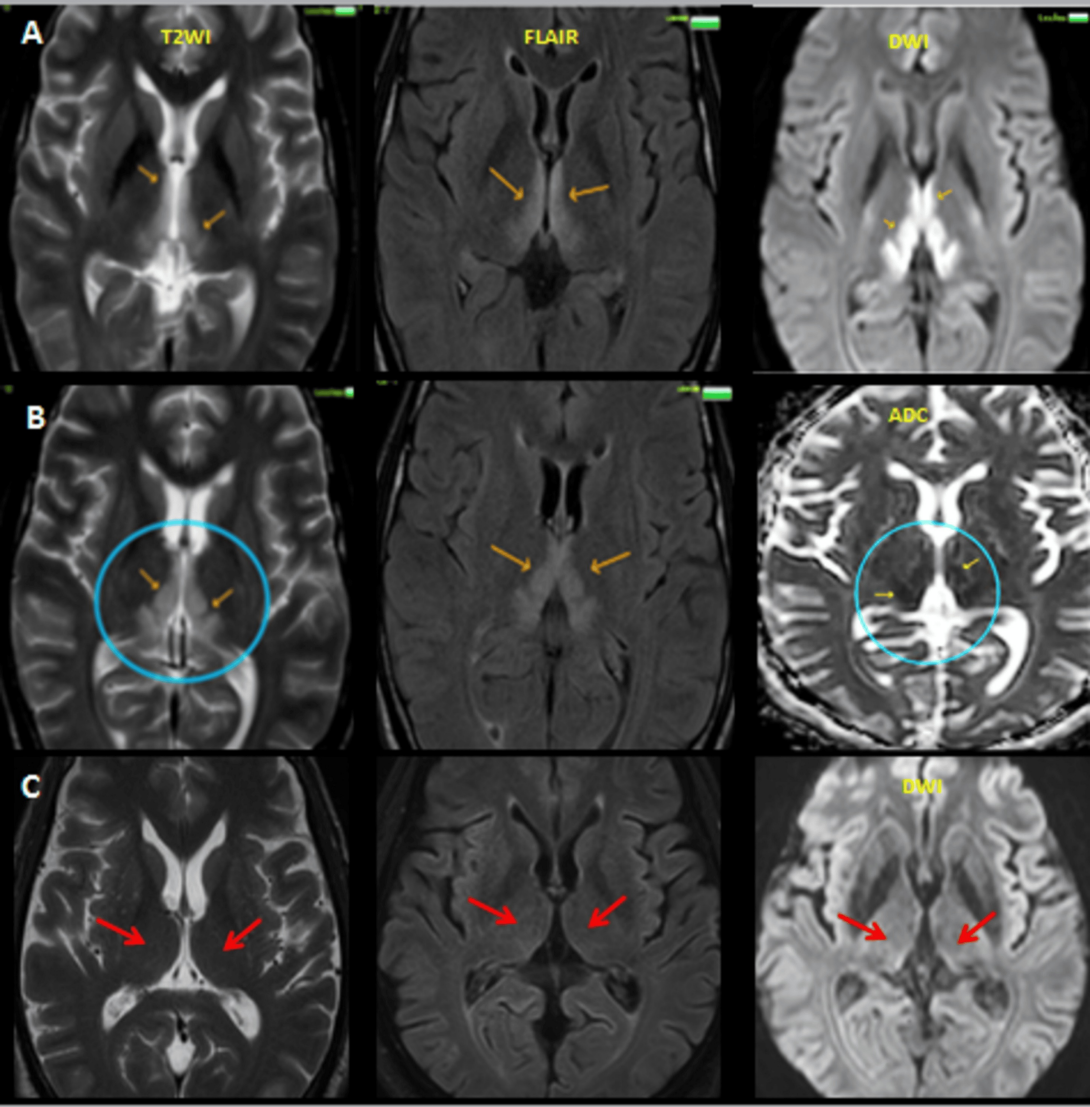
MRI brain of a 58-year-old male who presented with altered sensorium, ataxia, and vomiting following percutaneous transluminal coronary angioplasty for ischemic heart disease. (A & B) Axial T2-weighted, FLAIR, DWI, and ADC images demonstrate symmetrical hyperintensities (orange arrows and circled areas) in the mammillary bodies, dorsomedial thalami, periaqueductal gray matter, and around the third ventricle with diffusion restriction, findings characteristic of acute WE secondary to prolonged parenteral nutrition. (C) Following thiamine supplementation, the patient clinically improved, and follow-up imaging showed resolution of previous abnormalities with normalized signal intensity, indicating radiological recovery (red arrows) MRI: magnetic resonance imaging, FLAIR: fluid attenuated inversion recovery, DWI: diffusion weighted image, ADC: apparent diffusion coefficient, WE: Wernicke’s encephalopathy
